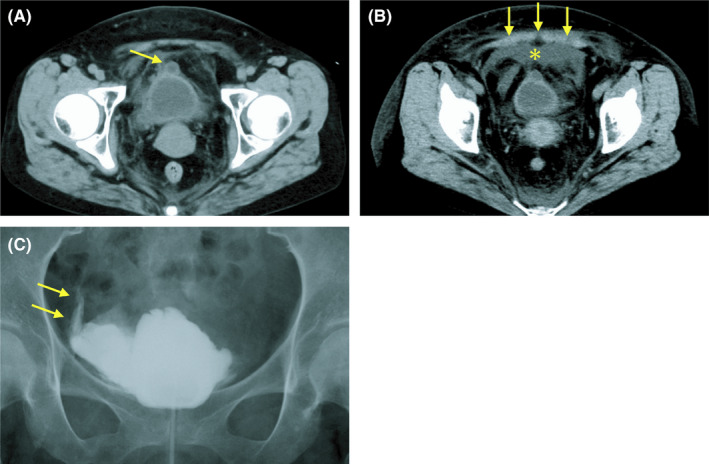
A, Initial CT scan showed bladder diverticulum (arrow) and thickened bladder wall with stranding in the surrounding fat. B, Repeated CT scan showed fluid collection in anteroventral portion of the bladder (asterisk) with thickening of pelvic peritoneum (arrows). C, Retrograde cystography showed contrast material moving outside the bladder (arrows)
